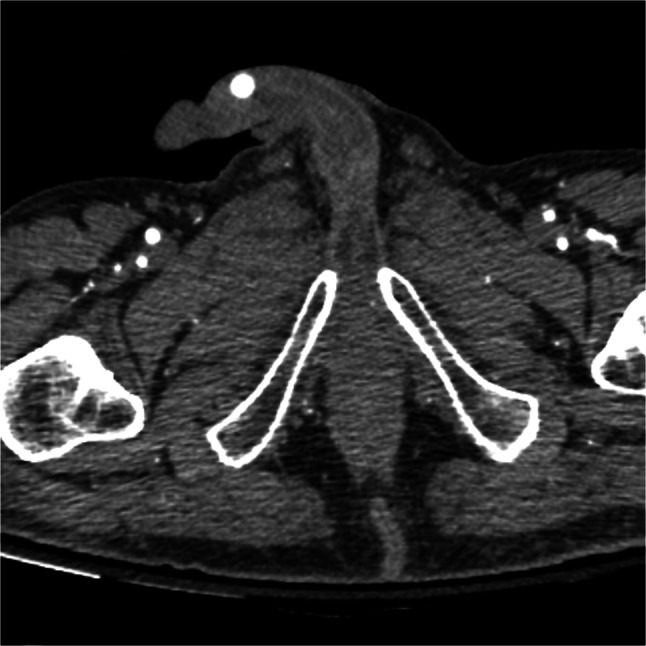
CT abdomen/pelvis demonstrating bead implanted into the subcutaneous tissue of the penis.
